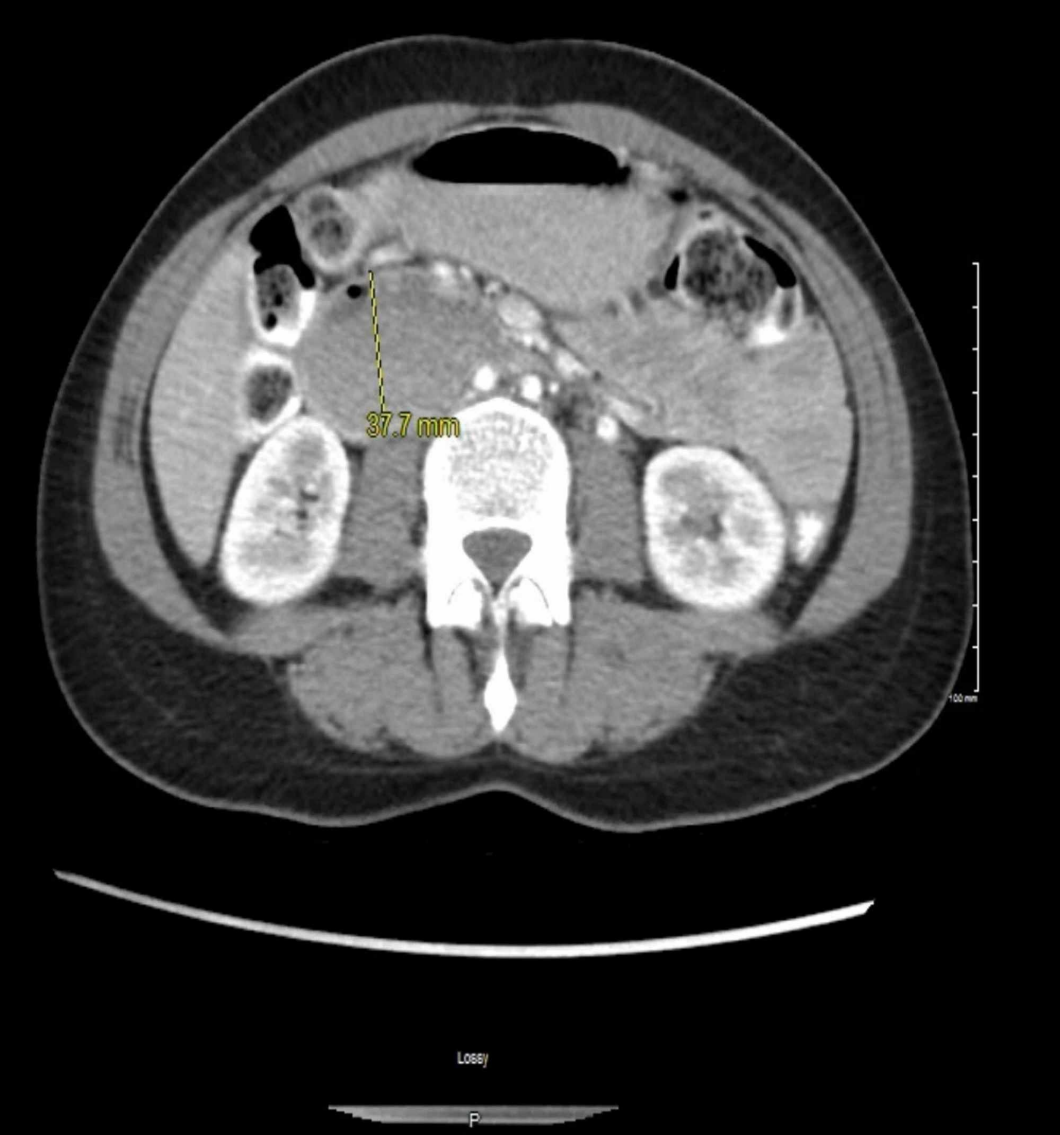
CT abdomen showing very little intra-abdominal fat, fluid-filled stomach, enlarged proximal duodenum measuring up to 37.7 mm
